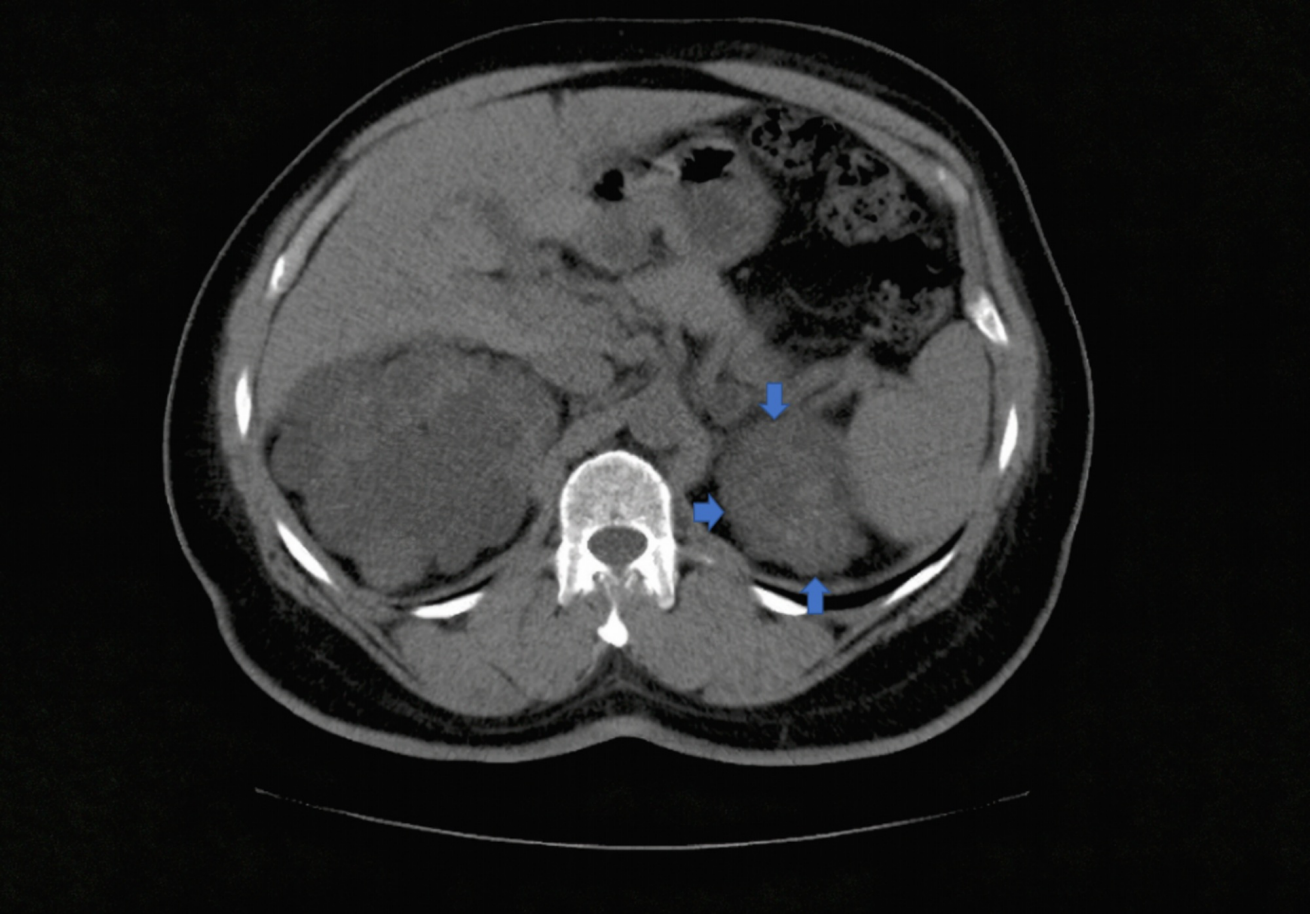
CT abdomen on transaxial section Blue arrows are marked to reveal the adrenal adenoma on the left side. CT: computed tomography
